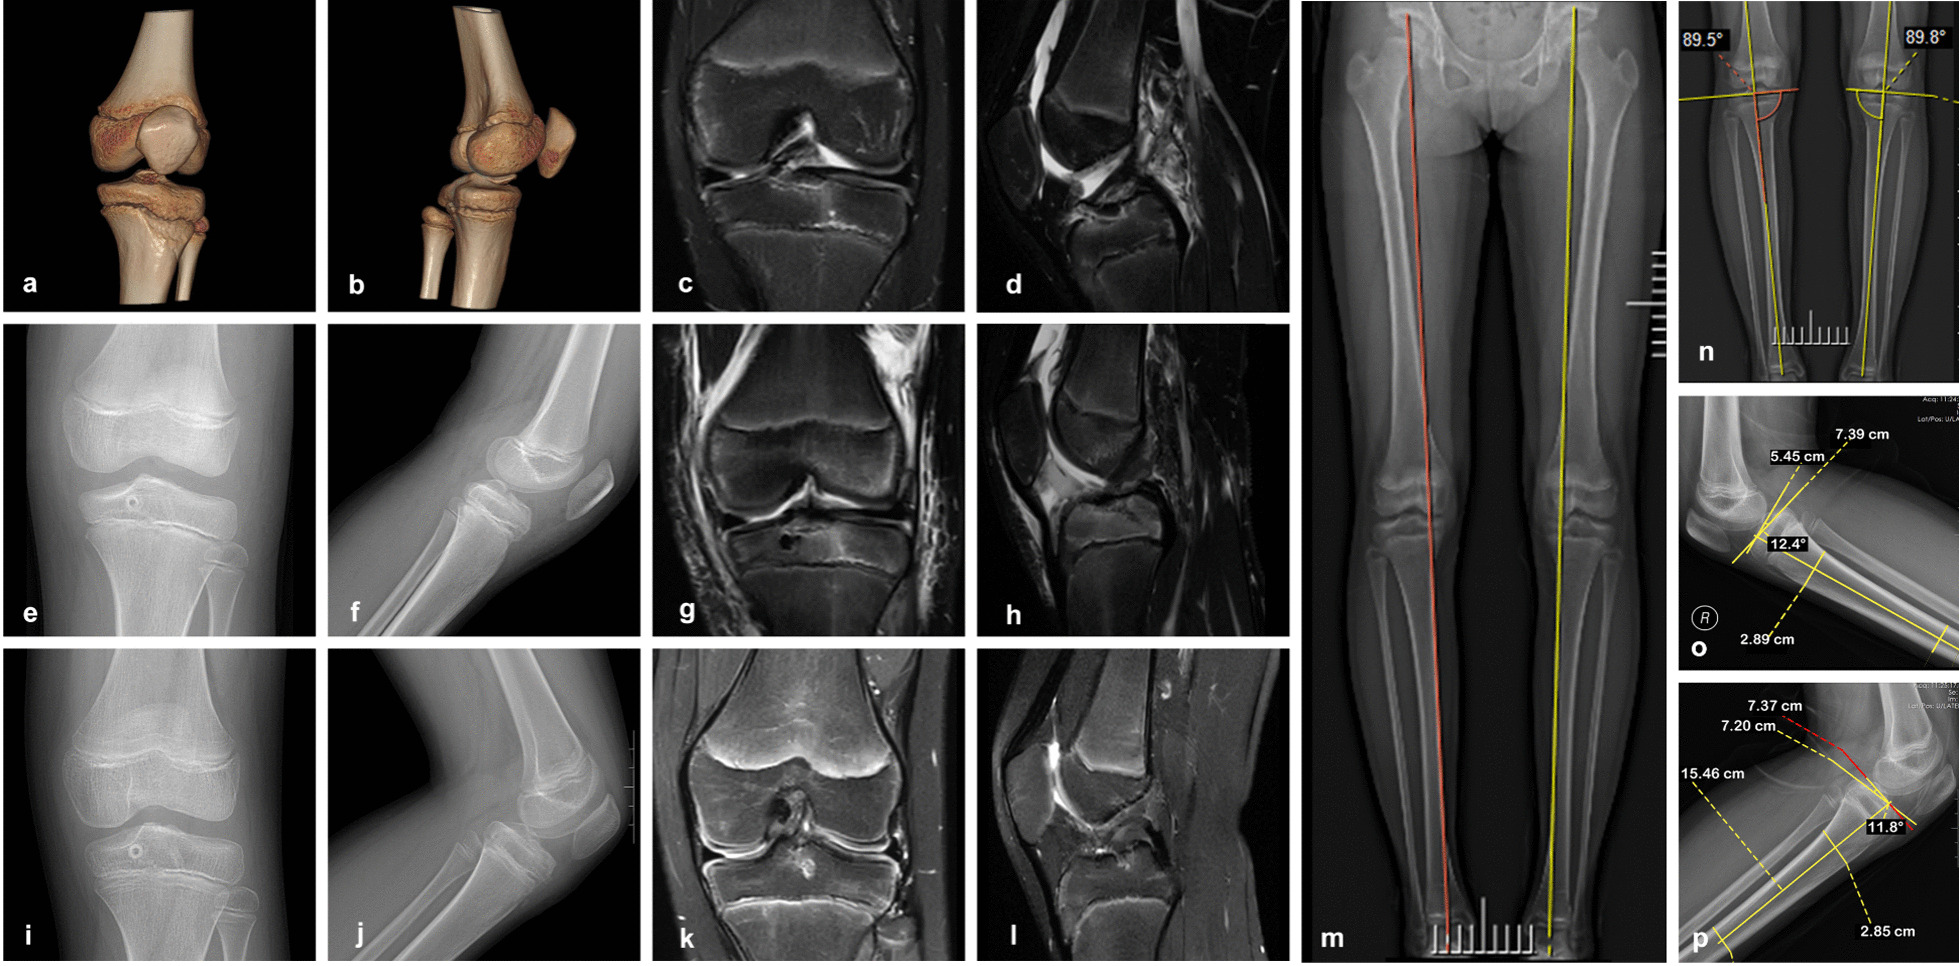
Female patient, nine years old, ACL tibial eminence avulsion fracture of the left knee. a–d Preoperative and images showing the type III tibial intercondylar eminence avulsion fractures. a, b Preoperative MRI. c, d Preoperative CT + 3D reconstruction. e–l Postoperative and follow-up images showed a good reduction of displaced tibial eminence fracture fragments and the restoration of the displaced fracture fragments. e, f X-ray on the third postoperative day. g, h MRI on the third postoperative day i, j X-ray on the first year postoperative. k, l MRI on the first year postoperative. m–p Postoperative and last follow-up X-ray images showed no difference in the length of the lower limbs and no angular deformities. m Long leg alignment on the third postoperative year showing the length of the lower limbs. n Long leg alignment on the third postoperative year showing the medial proximal tibial angle (MPTA). o, p Postoperative x-ray showing posterior tibial slope (PTS)
